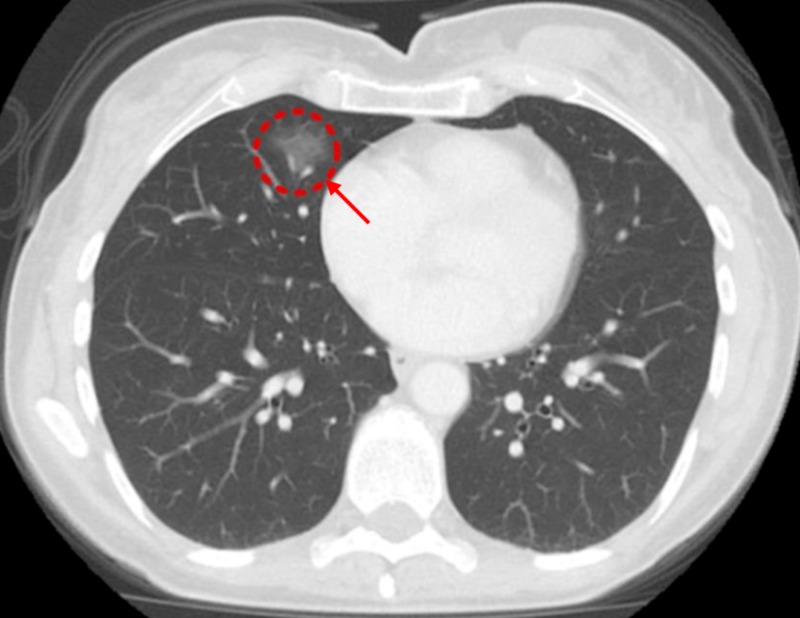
Initial computed tomography (CT) of the abdomen and pelvis with contrast showing partial visualization of ground-glass opacity (GGO) in the right middle lobe (RML)
